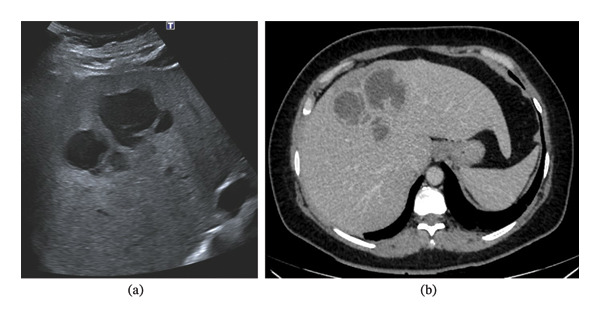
(a) On the day of admission, an abdominal ultrasound revealed three adjacent cystic formations in the hepatic dome, the largest measuring 4.8 cm with a lobulated contour, thickened wall and some septa. (b) On Day 1 after admission, an abdominal contrast‐enhanced CT showed a liver lesion affecting Segments IV and VIII measuring 9 × 8.5 × 5.5 cm.
